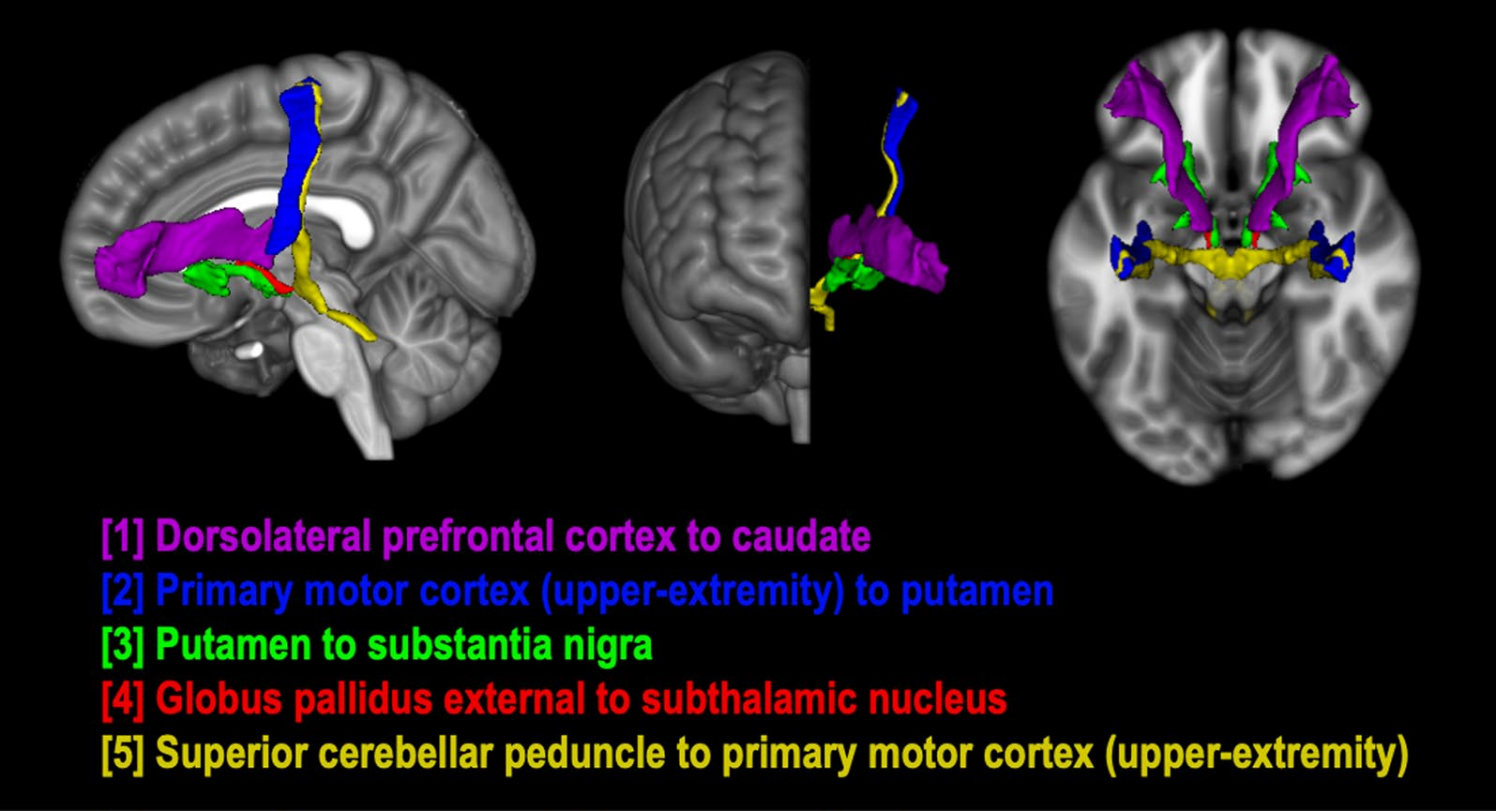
Basal ganglia and cerebellar white matter tracts of interest. Three-dimensional rendering of white matter tract templates depicted from lateral (left), anterior (center), and superior (right) viewpoints. Tracts between the following brain regions were included: (1) Dorsolateral prefrontal cortex to caudate—purple; (2) Primary motor cortex (upper-extremity) to putamen—blue; (3) Substantia nigra to putamen—green; (4) Globus pallidus externa to subthalamic nucleus—red; (5) Superior cerebellar peduncle to primary motor cortex (upper-extremity)—yellow. These tracts were subdivided along the midline into left and right hemispheres for diffusion MRI analyses
